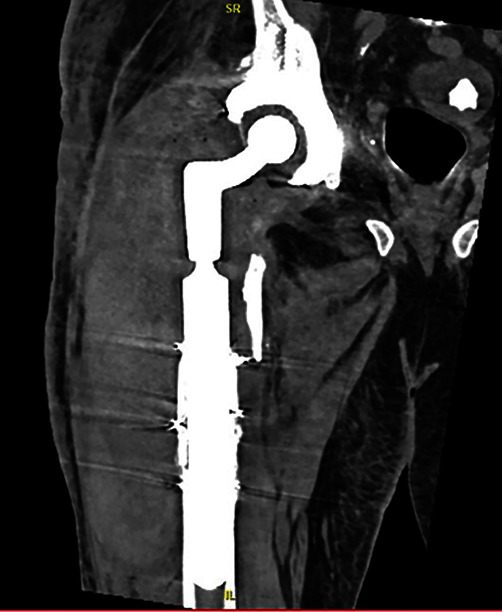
Computed tomography scan for right thigh swelling, revealing a 11.5 cm anteroposterior × 11 cm mediolateral × 33 cm craniocaudal hematoma.
